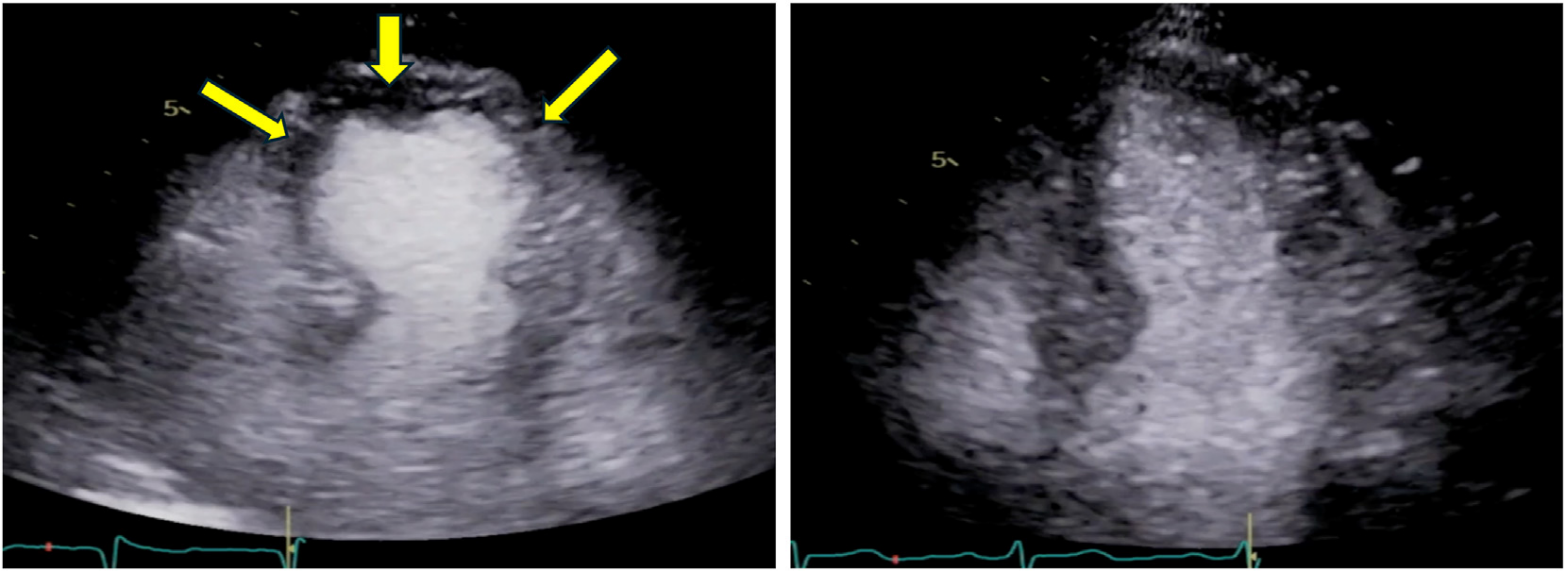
Echocardiogram demonstrating TCM. (*Leftpanel*) An apical 4-chamber view of a 2-dimensional echocardiogram of a patient with TCM with classical apical ballooning and hyperdynamic basal wall contraction of the left ventricle (*yellow arrows*). (*Right panel*) An apical 4-chamber view of a 2-dimensional echocardiogram with normal left ventricular systolic function. Clips are courtesy of the echocardiography laboratory of St Michael’s Hospital (Toronto, Ontario, Canada) and used with permission.
